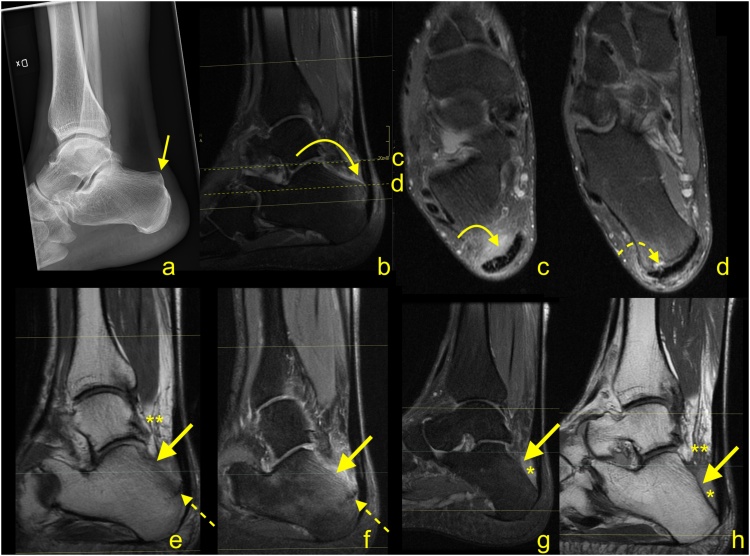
The postoperative complications for surgery to treat Haglund's syndrome. A 48-year-old patient presented with pain of the Achilles tendon insertion. X-ray (a) showed prominence of the supero-posterior part of the calcaneus (straight arrow). MRI (b, c and d) performed 3 weeks later revealed inflammation in the retrocalcaneal bursa, bone marrow edema in the bony prominence (curved arrow), and a cyst in the calcaneus (dashed curved arrow). In the postoperative period, the patient complained of pain in the heel, which was different than before the operation. The postoperative MRI (e, f, g and h) showed an incomplete fracture of the calcaneus (dashed arrow marks fracture line) near the Achilles tendon insertion and in the inferior part of the postoperative defect of the calcaneus (thick arrow). Postoperative reactive changes in Kager's fat pad (). Postoperative fluid collection is visible between the calcaneus, Achilles tendon, and Kager's fat pad (*). A- the lateral projection of the ankle joint, b, c and d- PD- weighted with fat suppression, e- PD-weighted, f and g- PD- weighted with fat suppression, h- PD- weighted.
